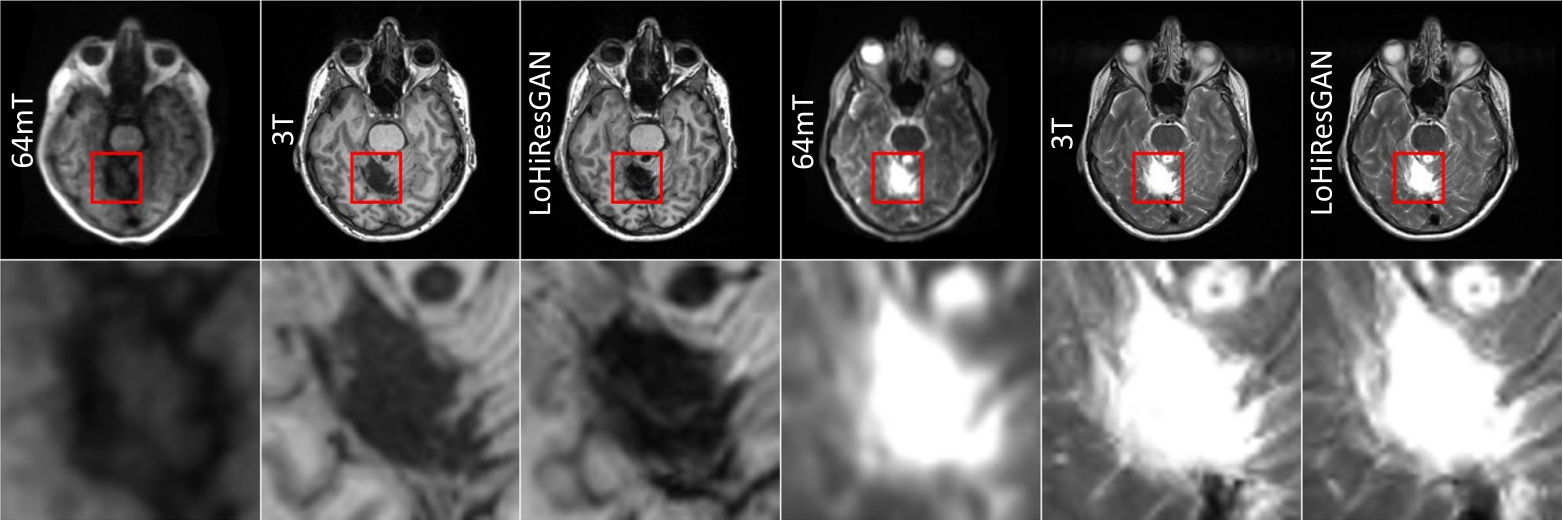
T1-weighted images (columns 1-3) and T2 weighted images (columns 4-6) using 64mT MRI scan, 3T MRI scan, and LoHiResGAN respectively in a 60-year-old female with focal encephalomacia. The second row of images displays the region of interest extracted from corresponding images.
